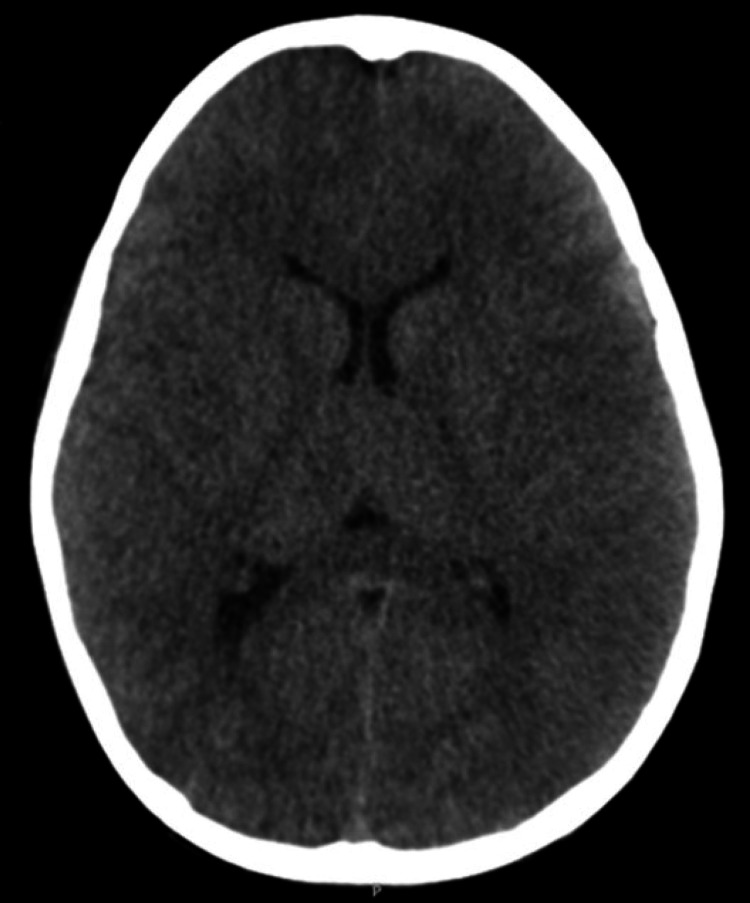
Head computed tomography scan revealing diffuse hypodensity of the brain parenchyma (diffuse dark areas), involving the cortico-subcortical parenchyma, the basal ganglia, and the thalamus.
